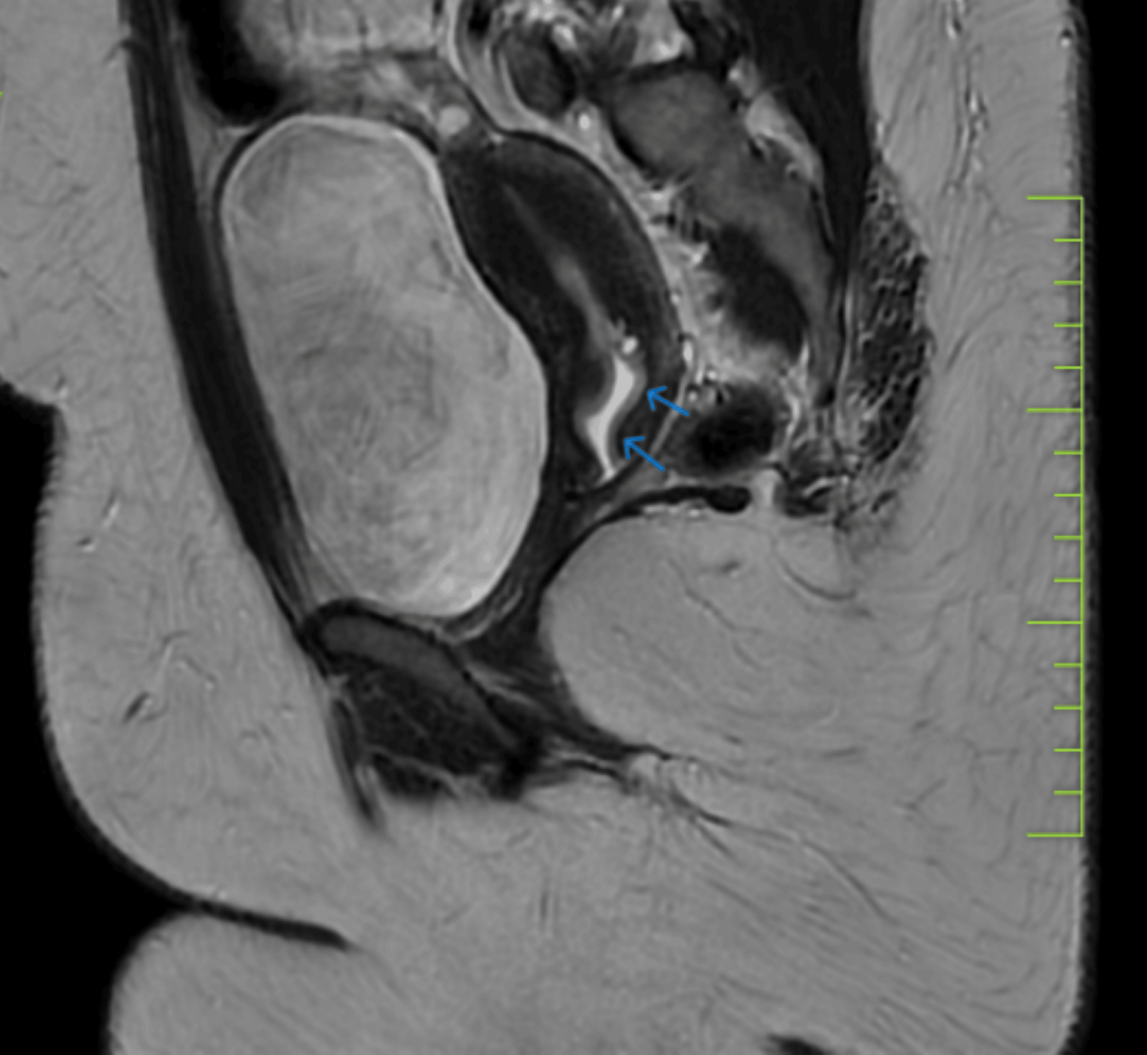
MRI pelvis in full bladder Overdistended bladder with a small amount of fluid within the endocervical canal.
